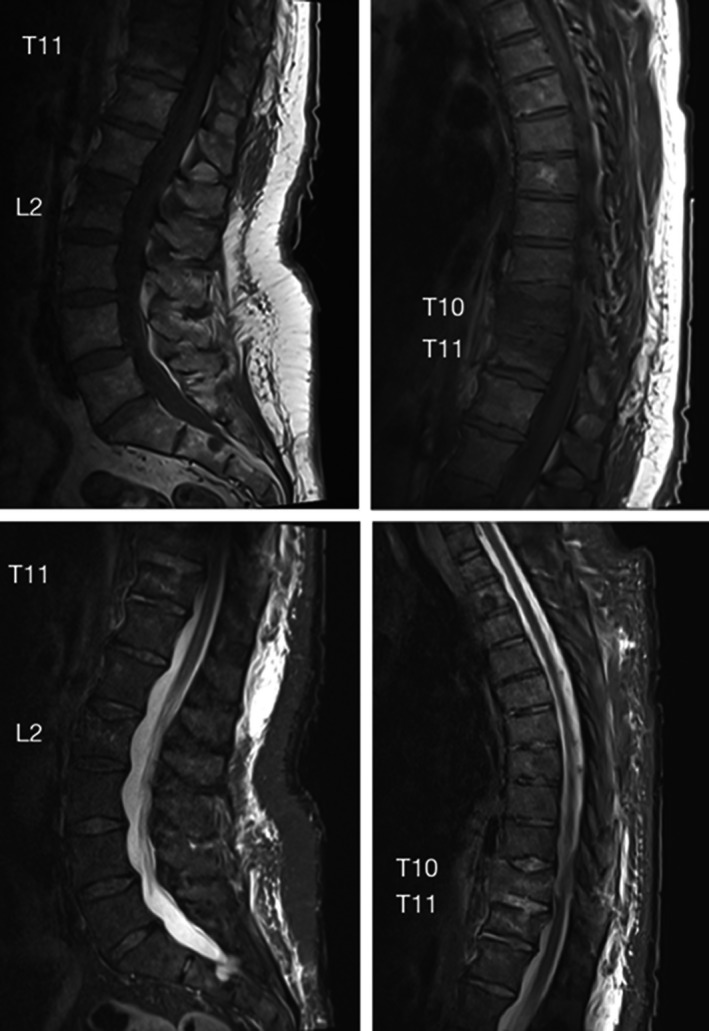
Preoperative MRI demonstrating some STIR changes at the T10, T11, and L2 vertebral bodies (bottom) with marked T1 hypo‐intensity in the same regions (top)
